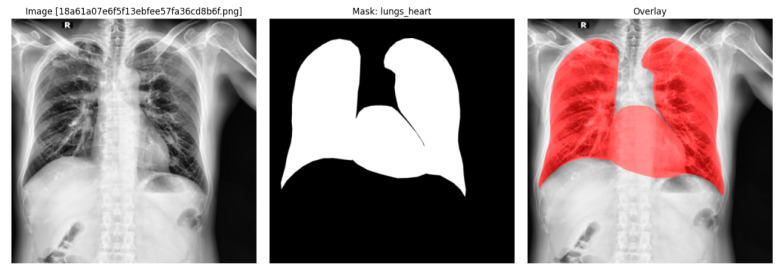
CheXmask anatomical masks used for post hoc localisation analysis. From (left) to (right): original chest radiograph, lungs and heart binary mask, and mask overlay. The red regions indicate the segmented lung and heart anatomical structures, and the letter “R” denotes the right side of the chest radiograph.
